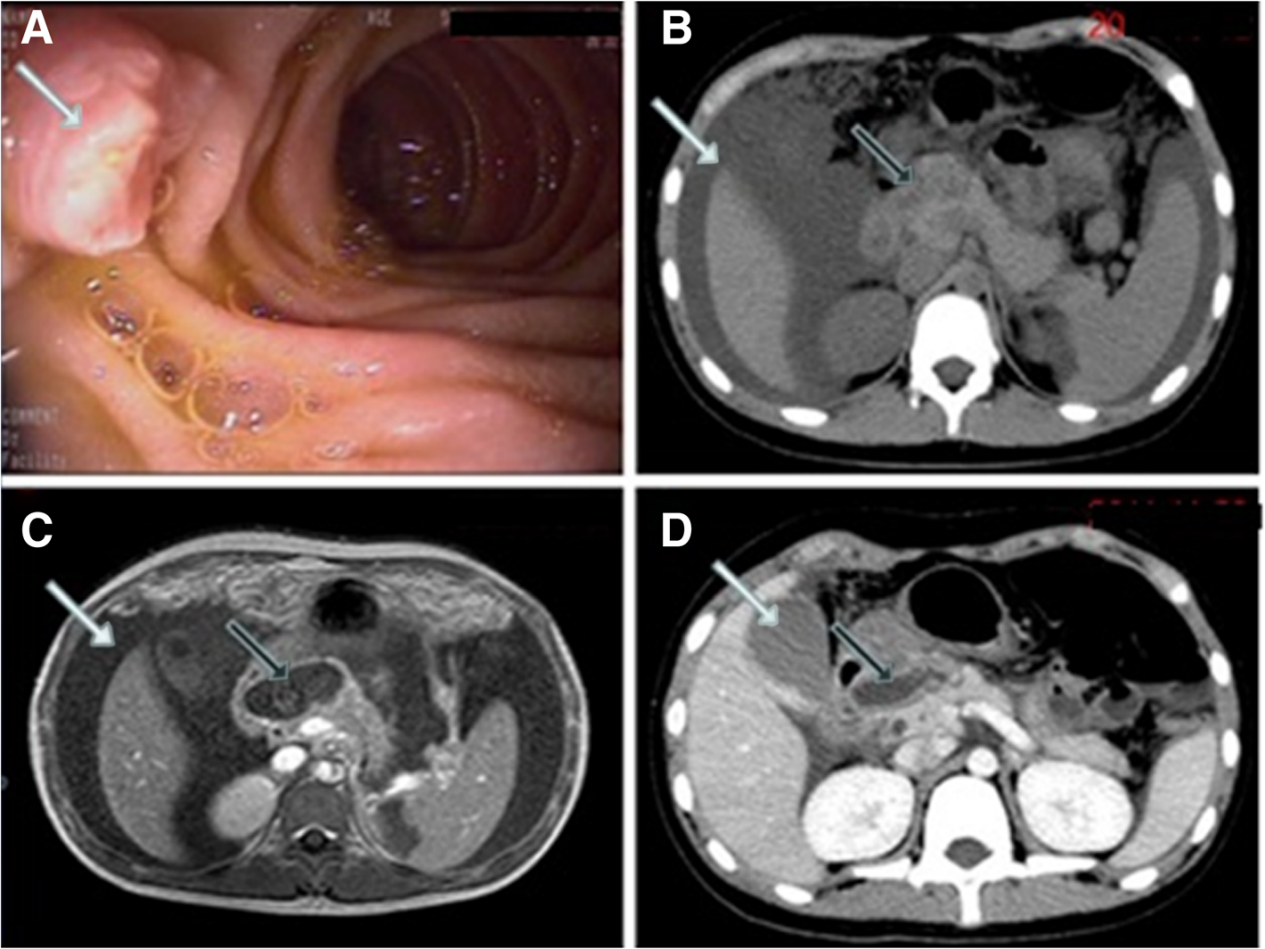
(A) Upper gastrointestinal endoscopy: the pancreatic papilla was swollen (white arrow). (B) Abdominal computed tomography (CT): A cystic lesion located between the descending portion of the duodenum and the head of pancreas (black arrow) and massive effusion signals in the abdominal cavity (white arrow). (C) Abdominal magnetic resonance imaging: A thick-walled cystic lesion with low signal intensity between the second part of the duodenum and the head of pancreas (black arrow), and massive effusion signals in the abdominal cavity (white arrow). (D) Abdominal CT: A well-delineated cystic lesion located between the descending portion of the duodenum and the head of pancreas. This lesion was not enhanced by the administration of an intravenous contrast: gadolinium (black arrow). The gallbladder was enlarged with high density in the bottom of the gallbladder (white arrow).
